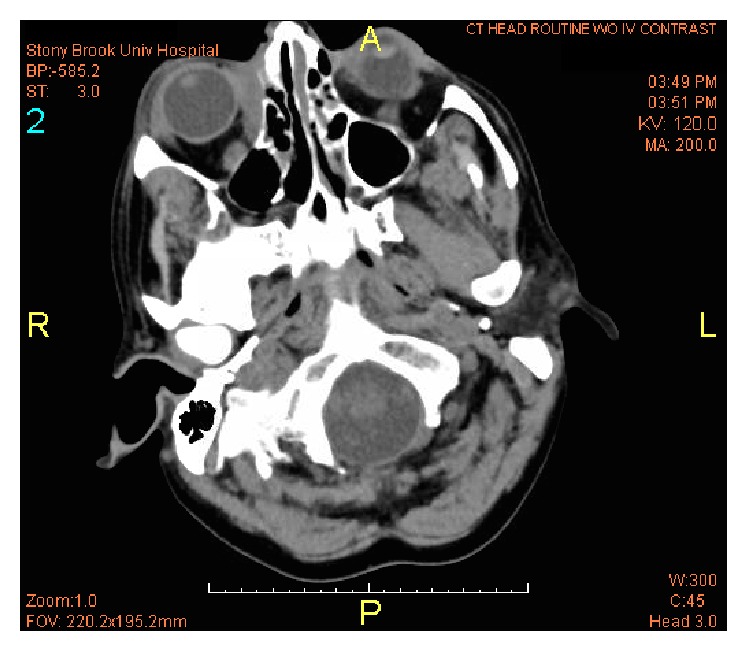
Head CT without contrast. After two cycles of R-CHOP therapy, interval CT scan revealed a significant decrease in size of right intraorbital mass lesion medially with small residual mostly in the medial extraconal space.
